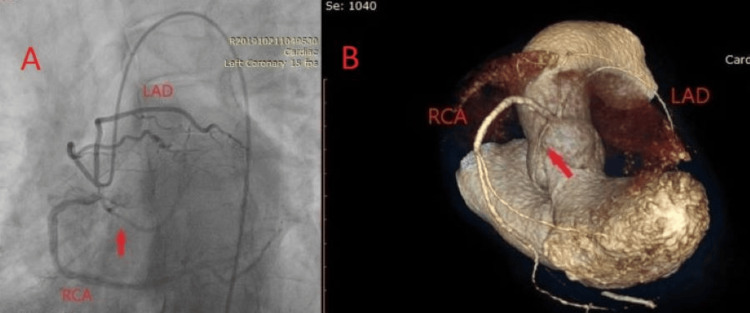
Case 3 A) Coronary angiography view showing LAD, RCA, and LCX originating from the right coronary sinus. The arrow indicates 50% stenosis of LCX. B) CCTA image showing RCA with a normal course, LAD originating from the right coronary sinus, while LCX (red arrow) has a retro-aortic course. CCTA: Coronary CT Angiogram; LAD: Left Anterior Descending Artery; RCA: Right Coronary Artery; LCX: Left Circumflex Artery
